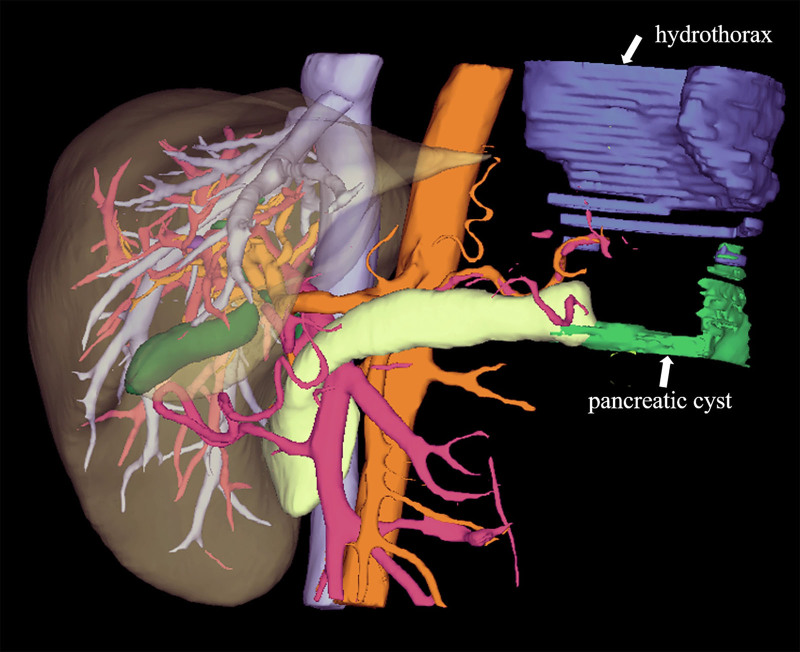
MRI three-dimensional reconstruction showing pancreatic cyst and pleural effusion caused by pancreatico-pleural fistula.
